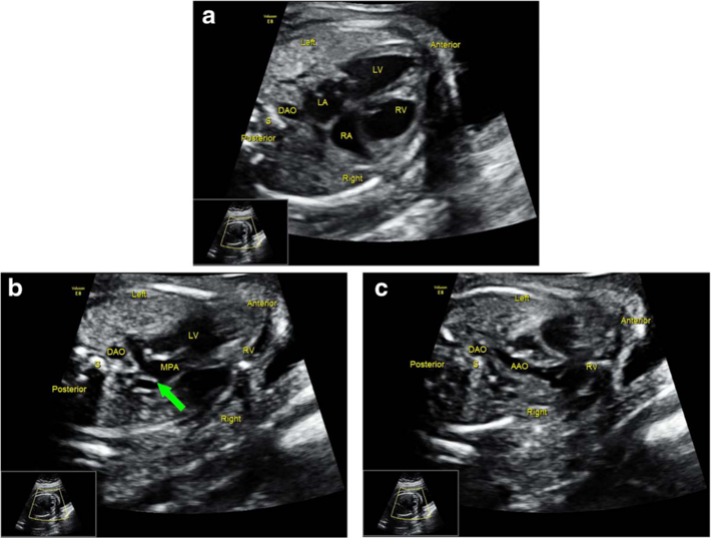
Sonographic images of a 22-gestational-week fetus diagnosed with TGA. The 4CV (a) shows four symmetric chambers with no obvious anomalies present. In the *left* (b) and *right* (c) outflow tract views, the connections of LV with MPA and RV with AAO are detected, respectively. Correct identification of the AAO and MPA was critical in making the diagnosis, which was accomplished by sonographers under Protocol B but failed under Protocol A. 4CV, four-chamber view; AAO, ascending aorta; DAO, descending aorta; LA, left atrium; LV, left ventricle; MPA, main pulmonary artery; RA, right atrium; RV, right ventricle; S, spine; TGA, transposition of the great arteries. The arrow indicates the bifurcation of the MPA
